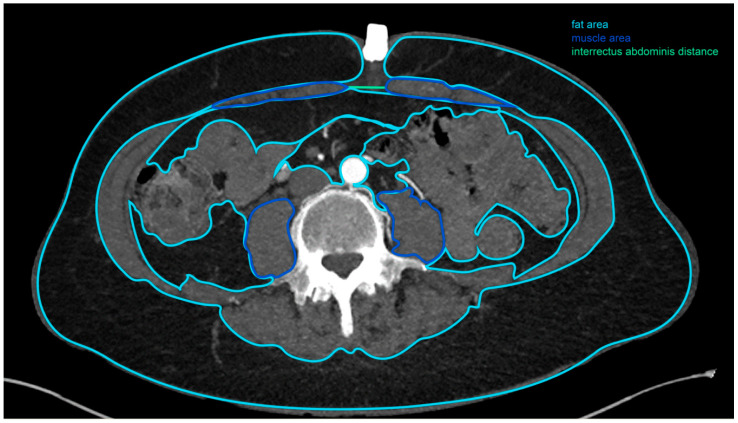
Computed tomographic angiogram (CTA) measurements.
